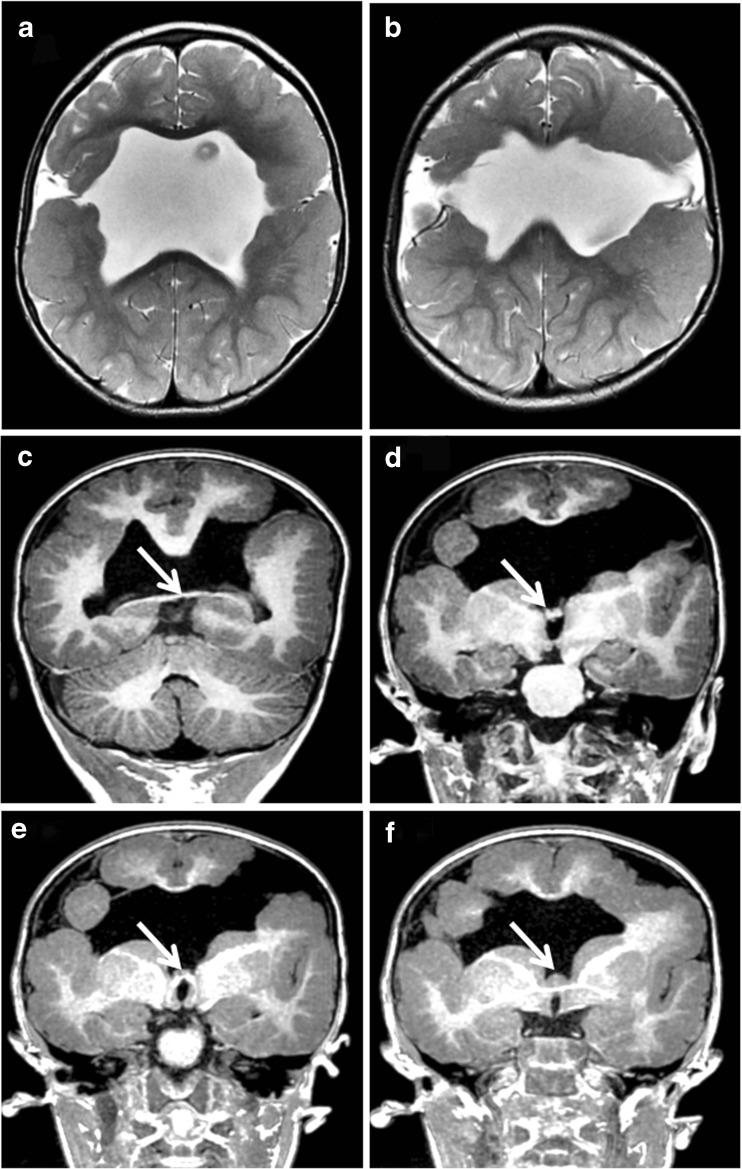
Bilateral schizencephaly (type 3). MR images of a 2-year-old child with global developmental delay. Axial T2-weighted (a, b) and coronal reconstructions from T1-weighted volume data (c–f) show bilateral CSF clefts with non-opposed borders lined with polymicrogyria. The septum pellucidum is absent and the fornices lie abnormally low (arrowed on c–f). Extensive cortical formation abnormalities were present in both hemispheres
